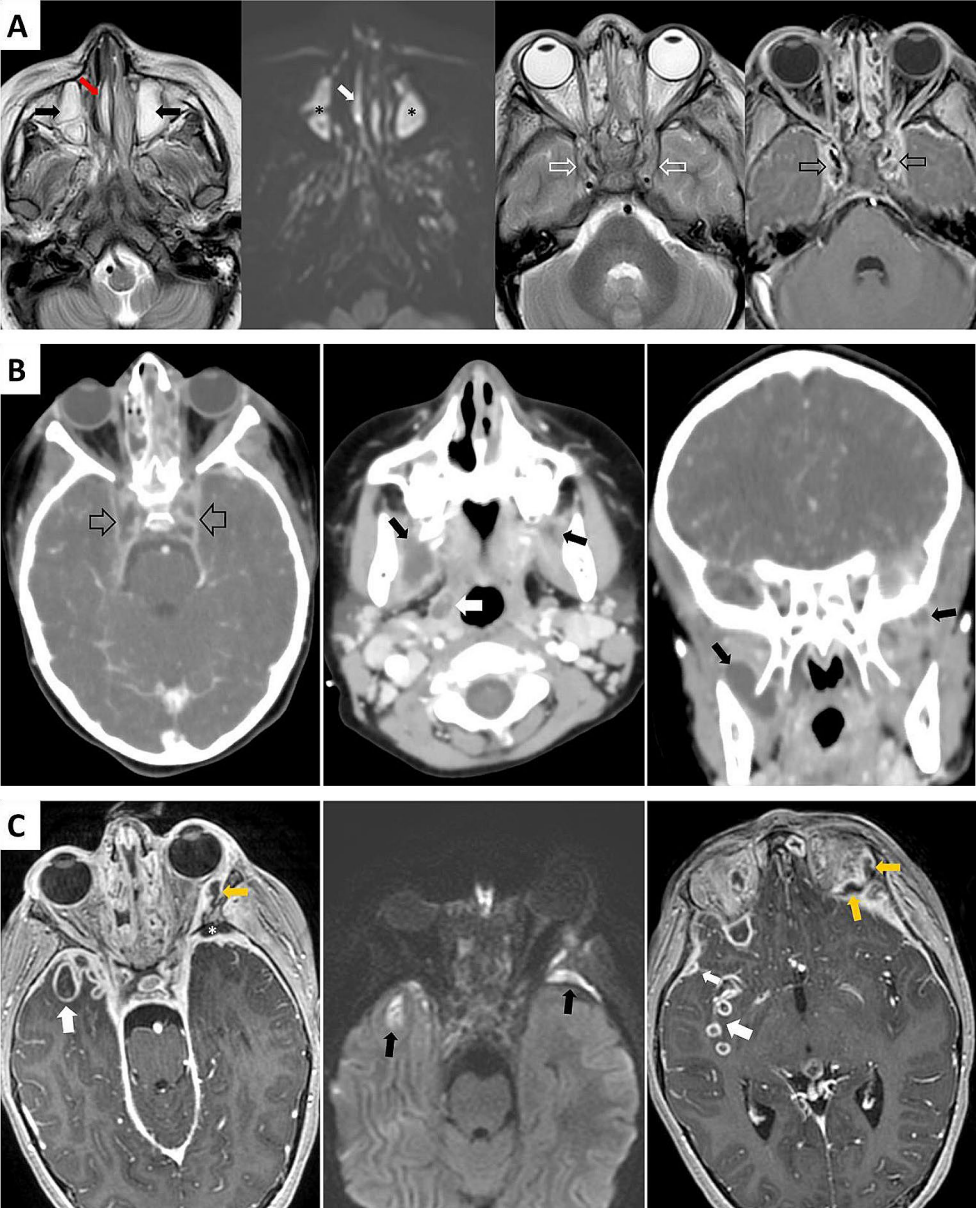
A: MR after bilateral VI cranial nerve paralysis debut. MRI T2 sequence revealed a sinusitis- pattern with pus occupying both maxillary sinuses (black arrows), showing restricted diffusion (asterisks). A right para septal abscess was observed (red arrow) showing restricted diffusion (white arrow). Bilateral cavernous sinus thrombosis was detected, it is characterized by enlargement and occupation of both cavernous sinuses on T2 sequence (white hollow arrows) and filling defects on T1 3D post-contrast sequence (black hollow arrows). B: CT 5 days after the first surgical procedure. Bilateral worsening of the cavernous sinus thrombosis. Large bilateral filling defects are observed (hollow black arrows). Both masticatory spaces are affected with abscesses in the right pterygoid muscle and the left temporal muscle insertion (black arrows). Also, a right peritonsillar abscess was present (white arrow). C: MR 1 week after the second surgical procedure. It demonstrated a left orbital abscess in the post-contrast T1 sequence (orange arrows). Intraparenchymal abscesses were observed in the right temporal lobe, also in the right insula (white arrows). Anepidural empyema contacted the left greater wing of the sphenoid bone (asterisk). Overall, the abscesses showed a restricted diffusion (black arrows) due to the presence of pus
